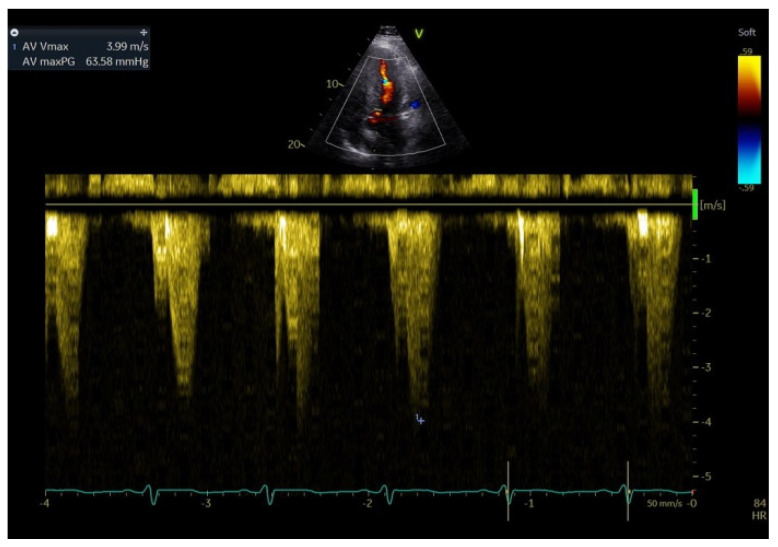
Continuous Wave Doppler in LV outflow tract measures a maximum gradient pressure of 63 mmHg.
